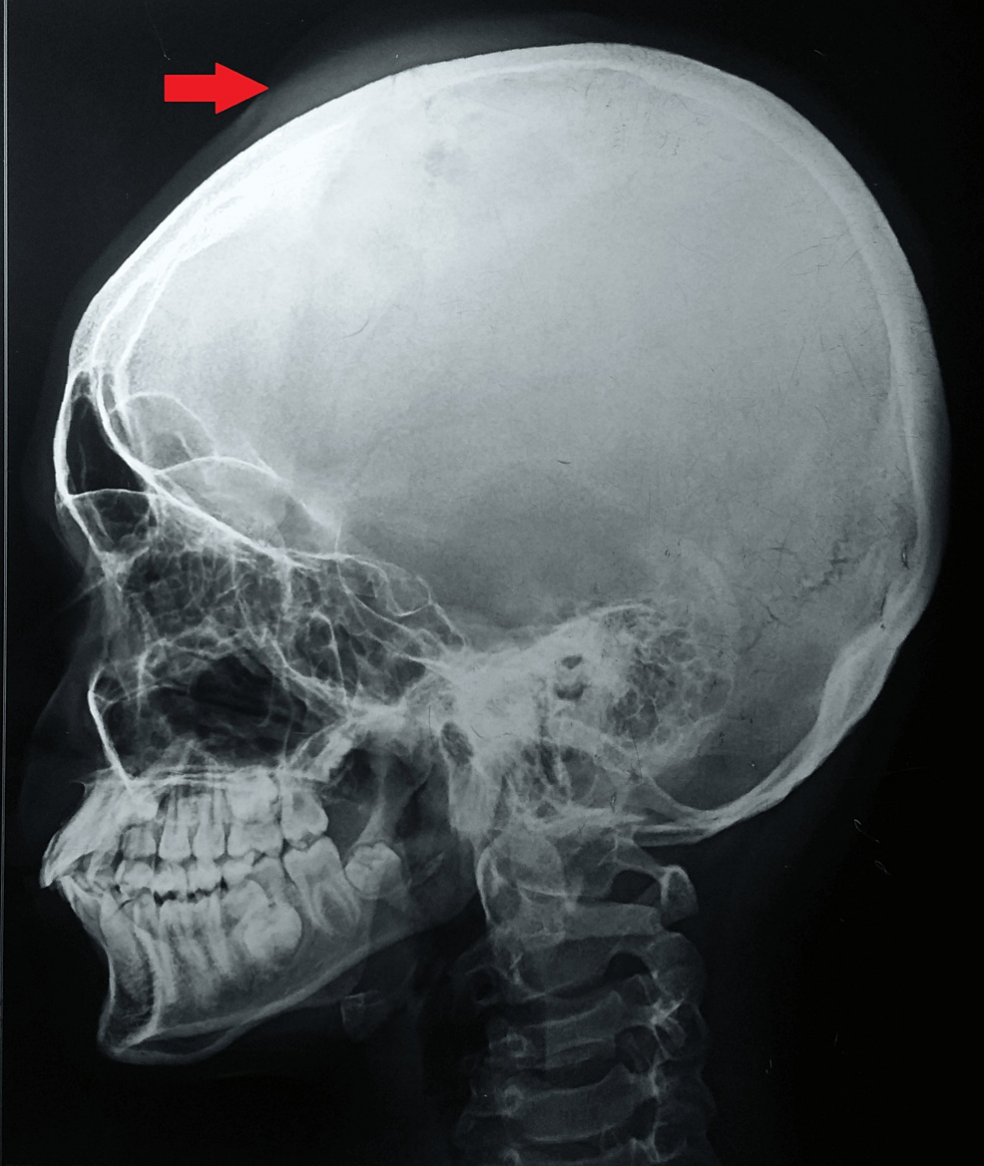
A plain radiograph of the skull (lateral view) suggestive of soft tissue swelling in the left parietal region
